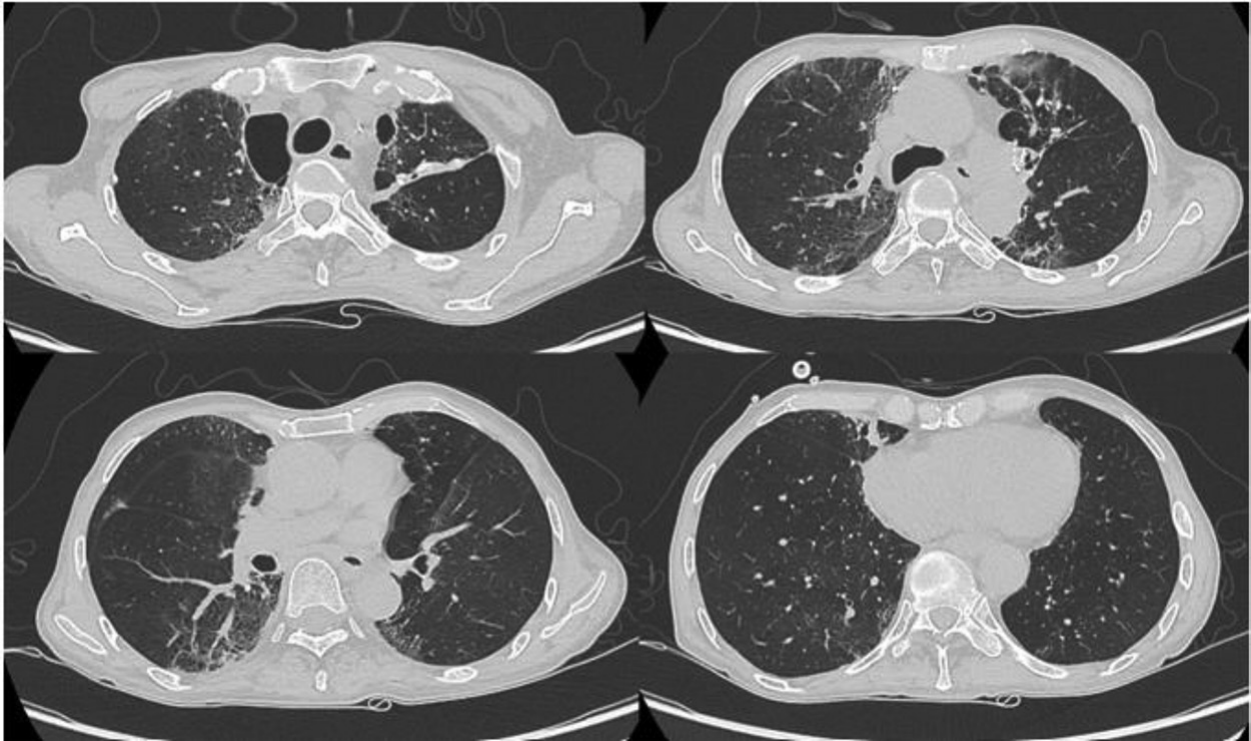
The chest CT scan after 1 week of intravenous ganciclovir and glucocorticoid therapy: Notable improvements in the same locations for comparison with the initial chest CT scan.
